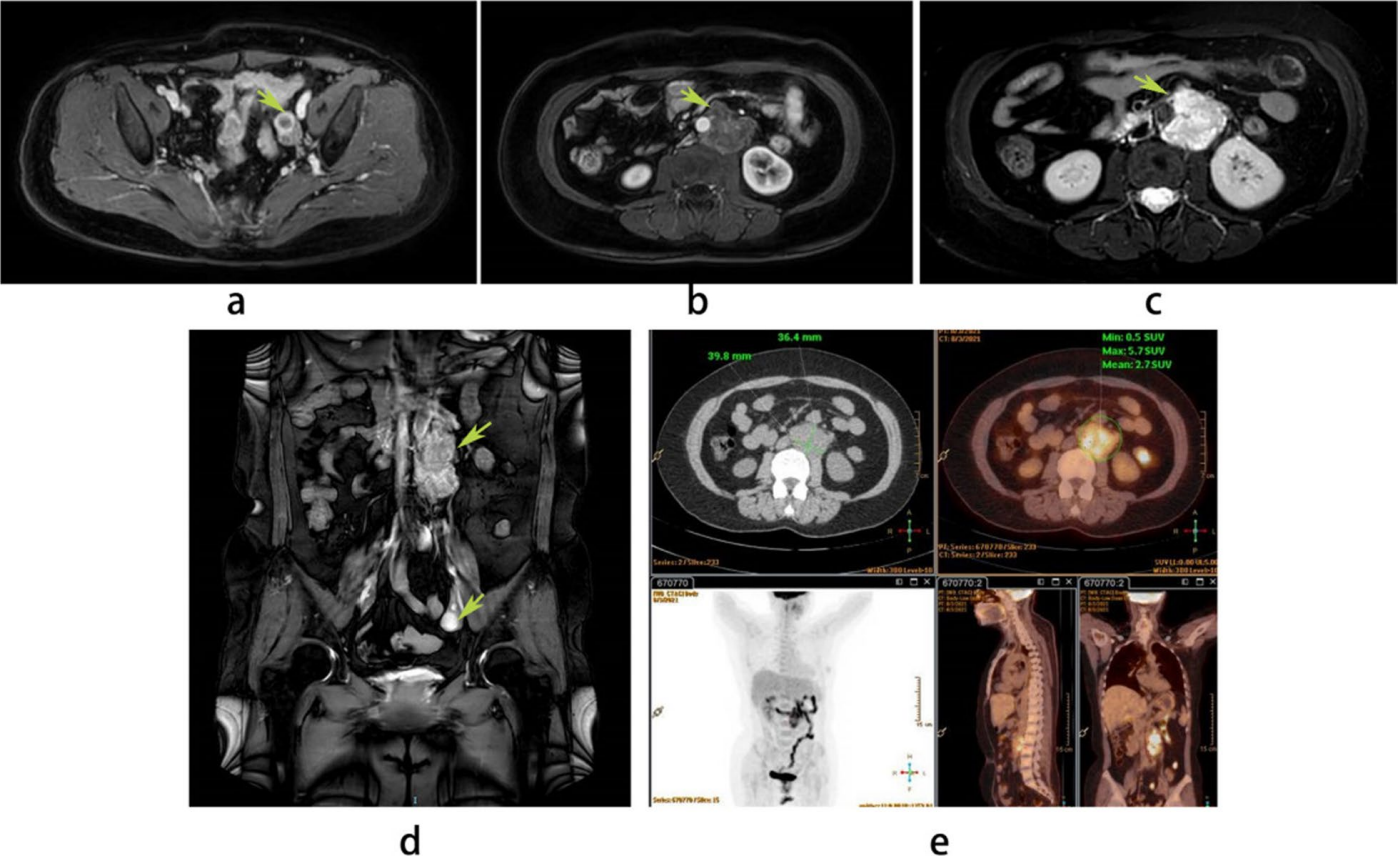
Findings on imaging of the patient. An MRI revealed that the left ovary was cystic and solid (a), and the irregular retroperitoneal mass at the lower margin of the first to the third lumbar spine measured approximately 75 × 42 × 37 mm^3^ (b–d). A PET-CT scan demonstrated that the lesion was on the left side of the retroperitoneal abdominal aorta about 70 × 40 × 36 mm^3^ in size with increased metabolic activity and unclear borders (e)
